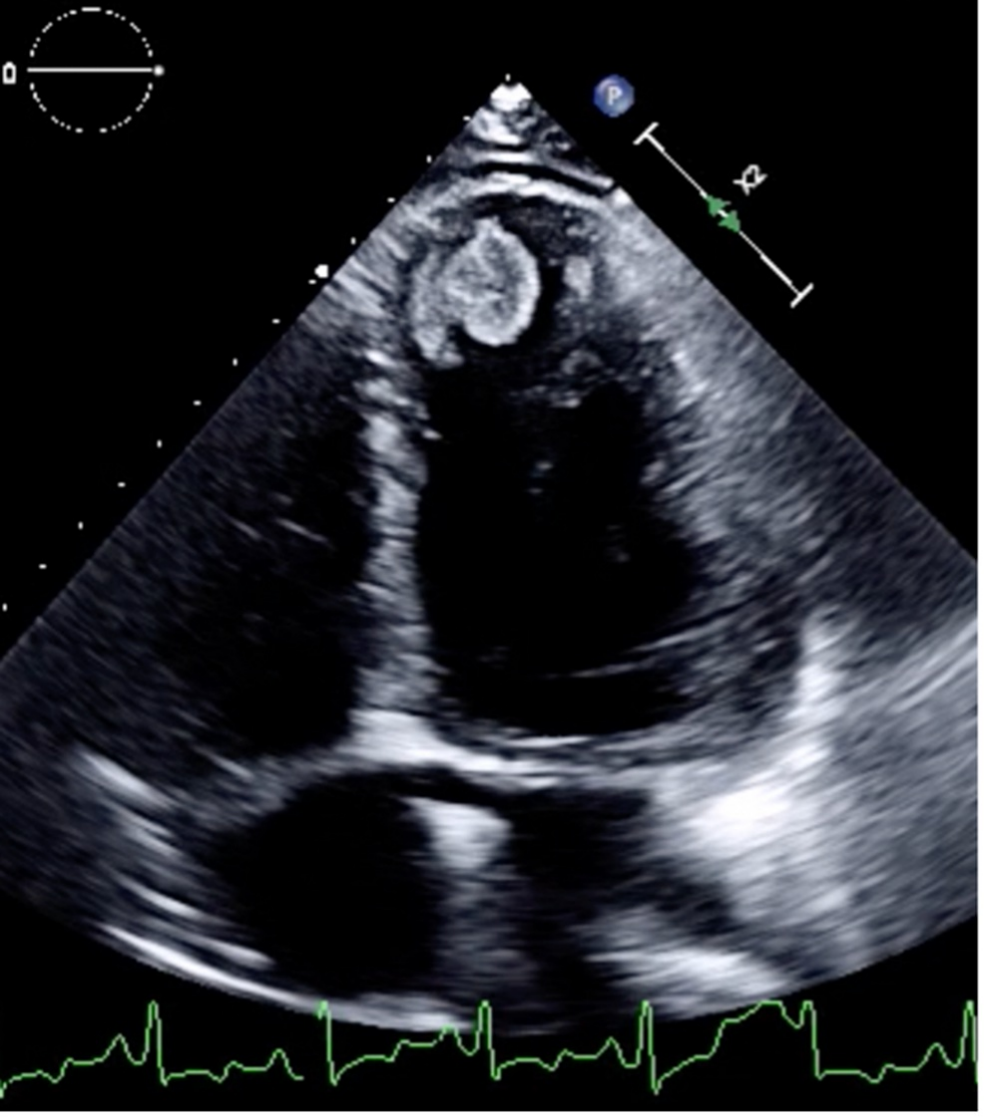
Apical Thrombus in Left Ventricle Echocardiography revealed a severely dilated left ventricle with a 2.1 x 1.2 cm apical density, suggestive of a thrombus and an ejection fraction of 20%.
